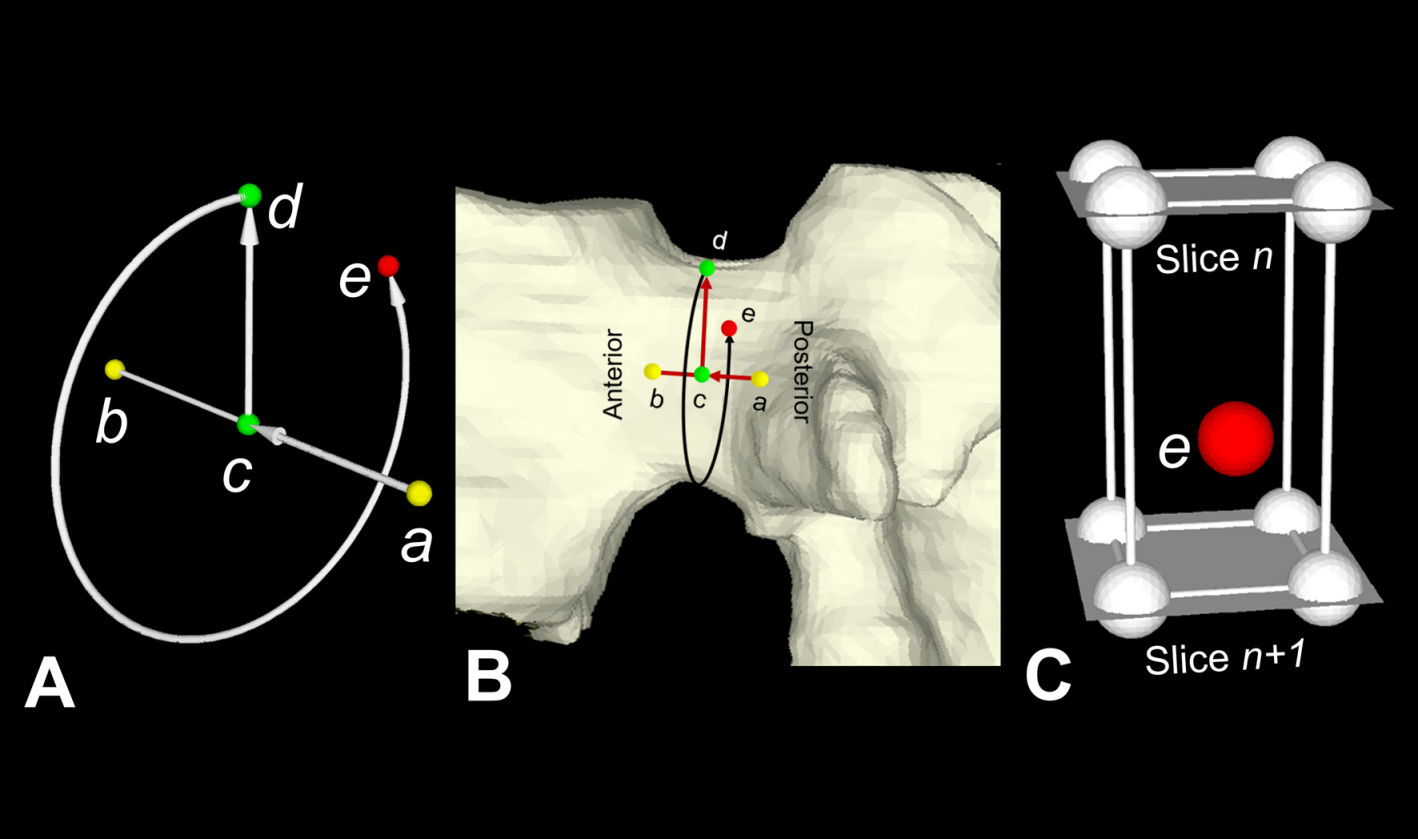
Determination of attenuation in Hounsfield Units (HU) at an arbitrary point in space using a cylindrical coordinate system with its longitudinal axis oriented along the pedicle’s long axis. (A,B) An arbitrary point *c* moves along a temporary pedicle axis connecting center points of the posterior and anterior pedicle ends (points *a* and *b,* respectively). Vector $$\overrightarrow {{{\varvec{cd}}}}$$ is perpendicular to vector $$\overrightarrow {{{\varvec{ab}}}}$$. The point *d* revolves around the pedicle axis and a point *e* is defined in the cylindrical coordinate system. (C) The HU value of the point *e* (red sphere) is calculated from HU values at 8 adjacent points (white spheres) in the 2 adjacent CT slices (slice *n* and slice *n* + 1) using a trilinear interpolation algorithm.
